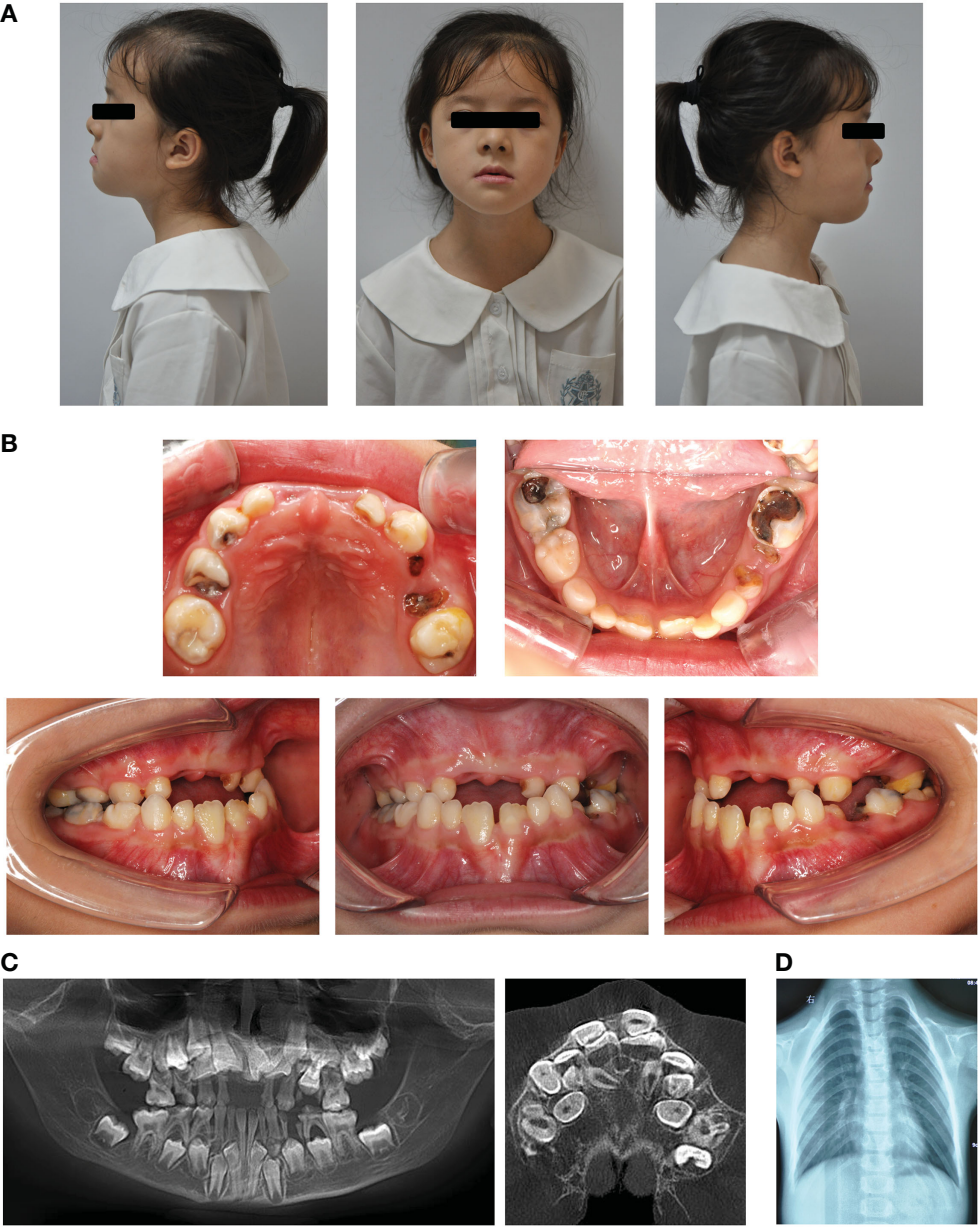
Typical clinical and radiological findings in the proband with CCD. (A) Front and profile photographs of the individual showing a concave face, hypoplasia of the maxilla, and a depressed nasal bridge. (B) Intraoral images showing enamel and dentin hypoplasia. (C) Panoramic view showing embedded permanent, supernumerary, and retention of deciduous teeth. (D) Chest radiograph showing hypoplasia of the left clavicle.
